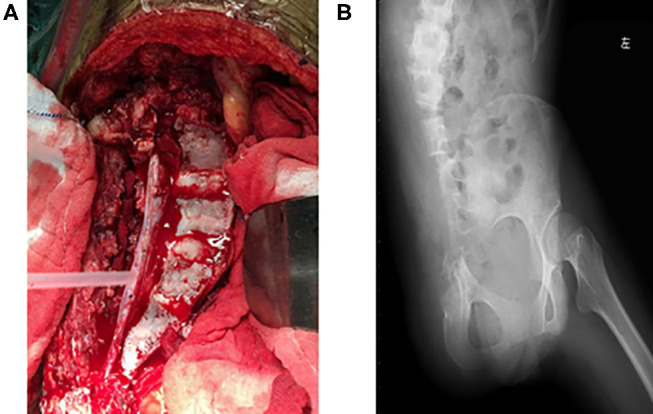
(A) Gross view of transverse resection of the sacrum and L5 during surgery. (B) Postoperative X-ray of the pelvis showing resection of the right ilium and semi-resection of the sacrum and L5 vertebra.
